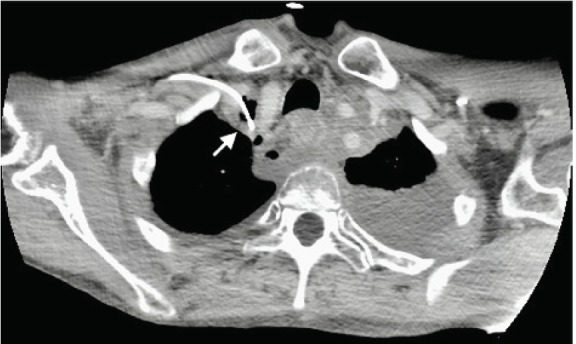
Transverse computed tomography thorax identifying peripherally inserted central catheter line perforation of right innominate vein, located superiorly and extraluminal in right upper mediastinum (arrow) with a large left pleural effusion.
